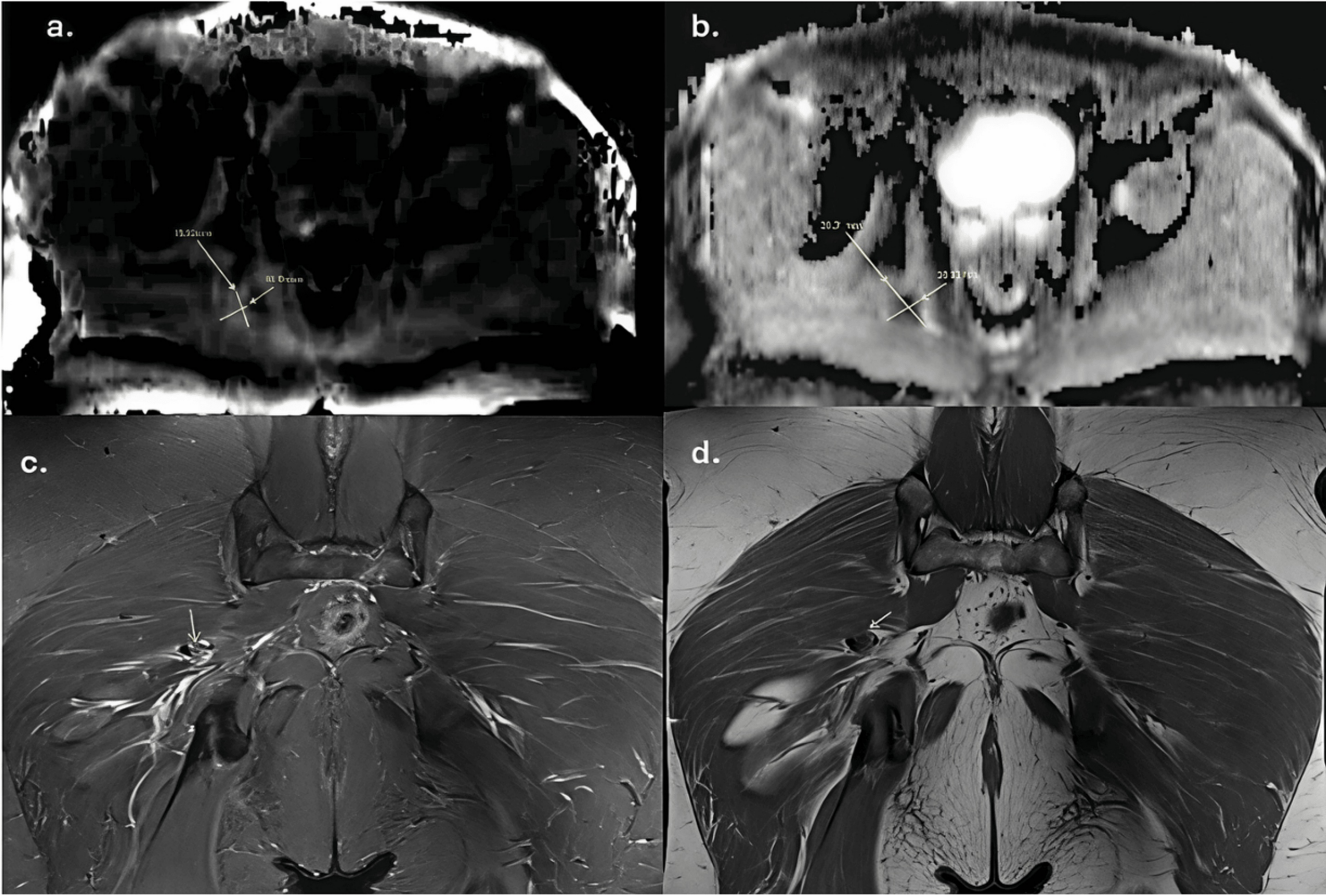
Contrast-enhanced magnetic resonance imaging Nodular lesion (arrows) demonstrating marked diffusion restriction, appearing hyperintense on DWI (a) with low signal values on the ADC map (b), hyperintensity on the STIR sequence corresponding to edema underlying the lesion (c), and isointensity to muscle on T1-weighted imaging (d). DWI: Diffusion-weighted imaging; ADC: Apparent diffusion coefficient; STIR: Short tau inversion recovery.
